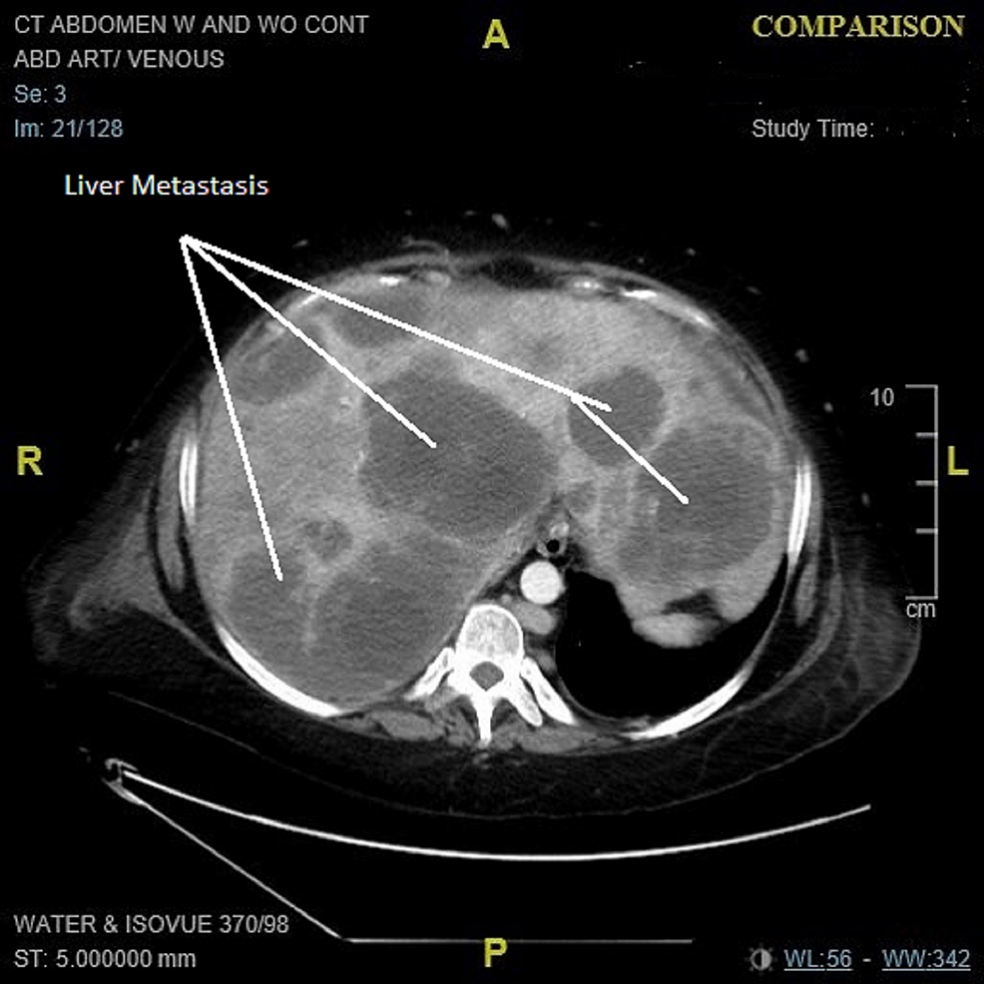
CT abdomen (axial plan): multiple liver metastasis.
